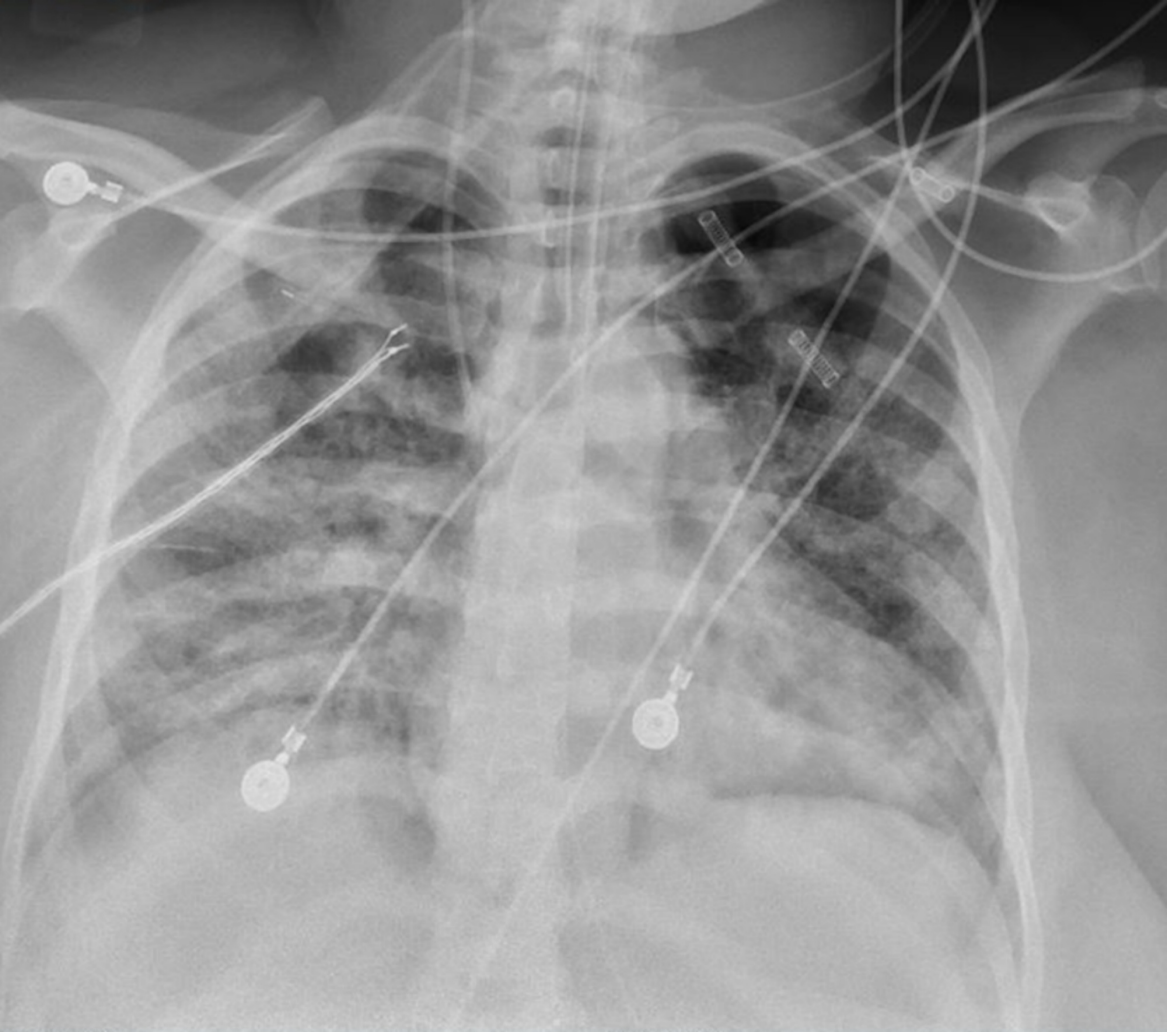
Chest radiography (CXR) on hospital day five showed diffuse heterogeneous airspace opacifications.
